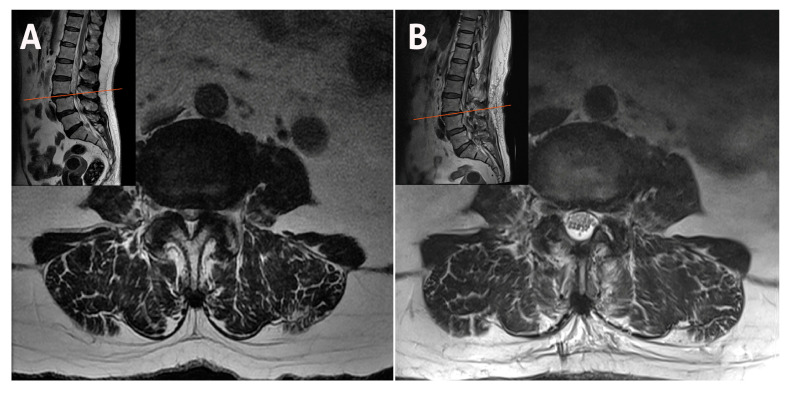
(A) MRI demonstrating spinal stenosis with vertically oriented facets at the L3–L4 level with grade 1 spondylolisthesis. (B) MRI image of a patient with spondylolisthesis post-decompression showing preservation of both facets. MRI, magnetic resonance imaging.
